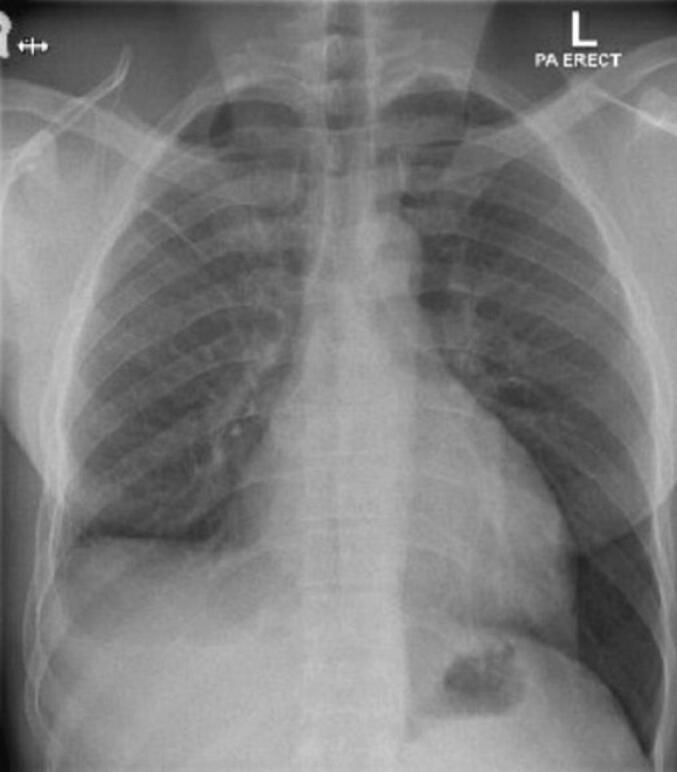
Chest X-ray on follow up 1 week later.
